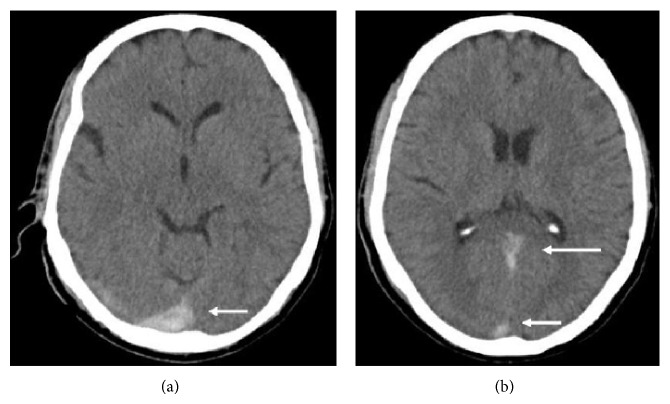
First CT scan brain without contrast showing suspicion of subarachnoid bleed. (a) Hyperdensity involving the posterior most portion of falx cerebri and extending to right (white arrow)-(trickling trail of blood); (b) hyperdensity extending along falx cerebri posteriorly (white arrow long) and marginally to the right (white arrow short).
